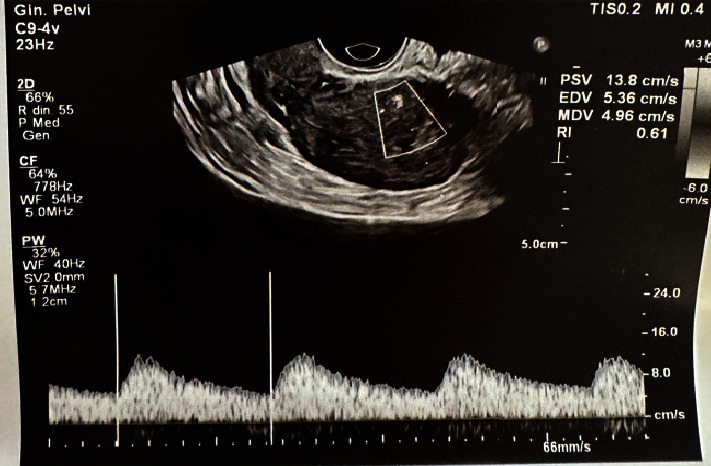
Transvaginal ultrasound check 30 days after hysteroscopy, which highlighted a PSV of 13.8 cm/s.
